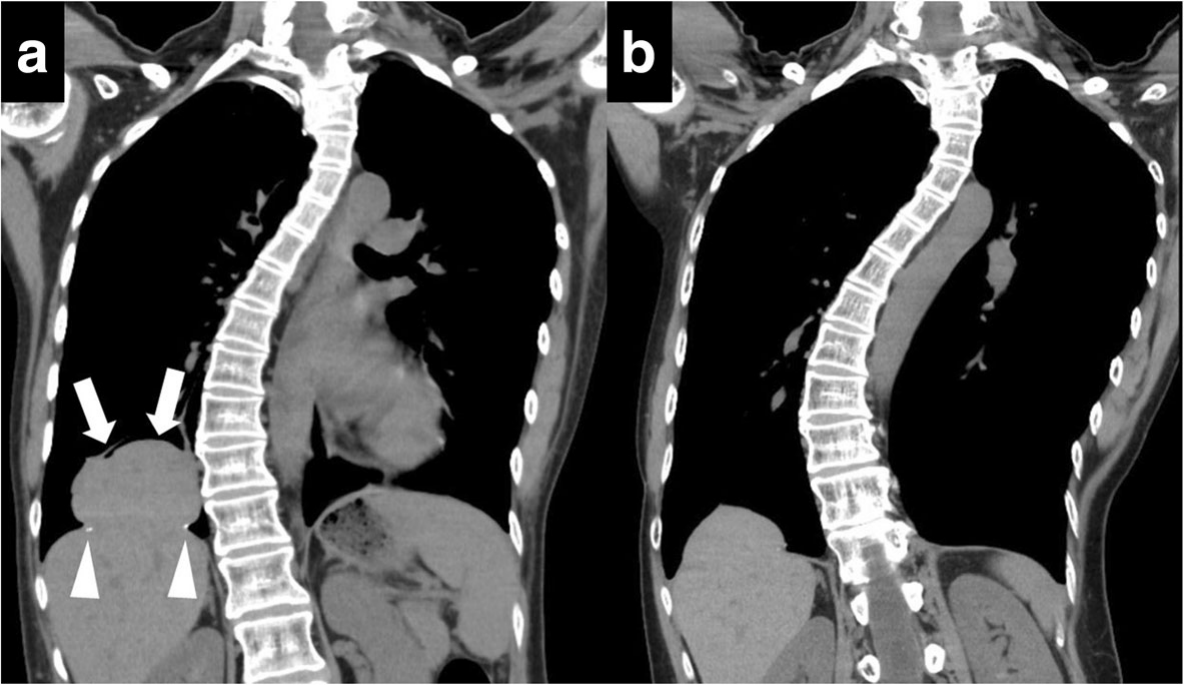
a Computed tomography revealed a right diaphragmatic defect with herniation of a part of the liver (6.6 × 4.5 cm) into the thorax (*white arrows*). Surgical staples were observed around the edge of the diaphragmatic defect (*white arrowheads*). b Computed tomography finding 3 years after the scan obtained in Fig. 1a. There has been no enlargement of the hernia
